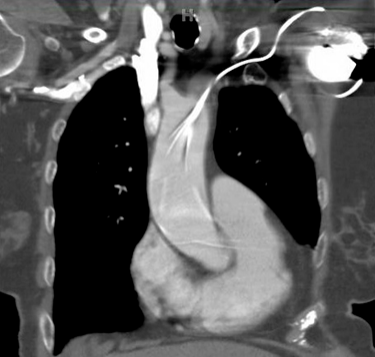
CT Chest Demonstrating Misplaced Single Lead Pacemaker
